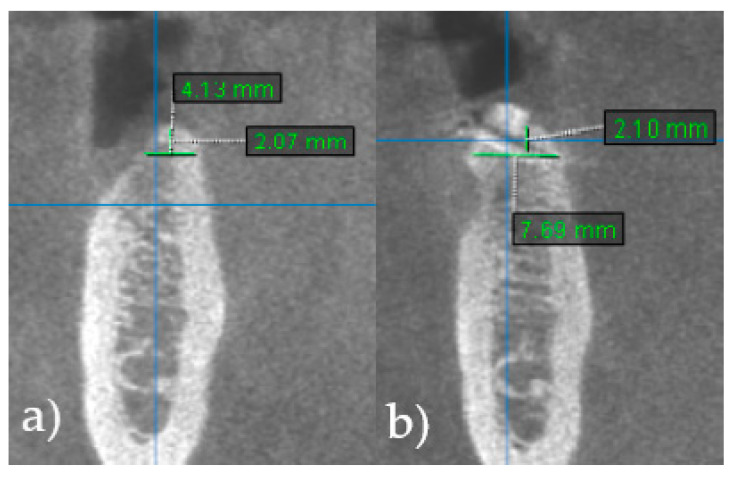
CBCT scans show horizontal dimension of the alveolar ridge. (a) Ridge width before (RWb) alveolar ridge augmentation surgery. (b) Ridge width 6 months after surgery (RWa), prior to implant placement.
